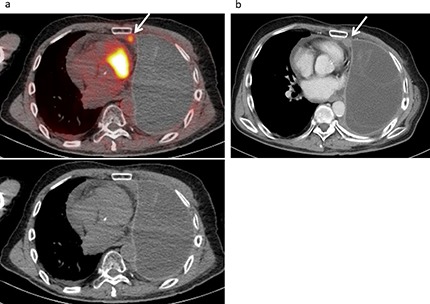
Local recurrence developed in a 67-year-old male following treatment for a malignant pleural mesothelioma, including neoadjuvant chemotherapy, left extrapleural pneumonectomy, radiotherapy, and chemotherapy. (a) FDG-PET/CT and CT findings showed low level mild FDG uptake (SUVmax 3.28) in the ipsilateral mediastinal pleura (arrow), suggesting local recurrence with a score of 4. (b) Contrast-enhanced CT revealed a tiny enhanced mass (10×11 mm) in the ipsilateral mediastinal pleura (arrow) with a score of 3 (false negative).
